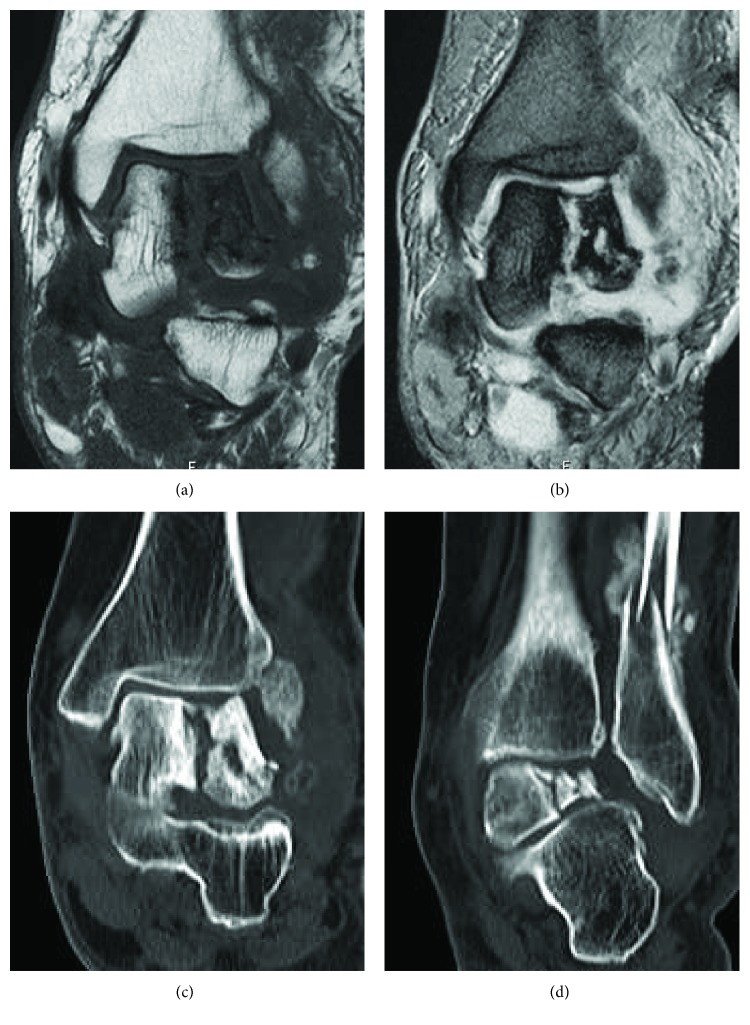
T1-weighted (a) and short-T1 inversion recovery (b) coronal magnetic resonance imaging of the left ankle showing a vertical fracture of the talar body and osteonecrosis of the lateral talar body fragment. Computed tomography images of the left ankle showing the comminuted fragments of the lateral talar body (c) and the fracture of the distal fibula with callus formation (d).
